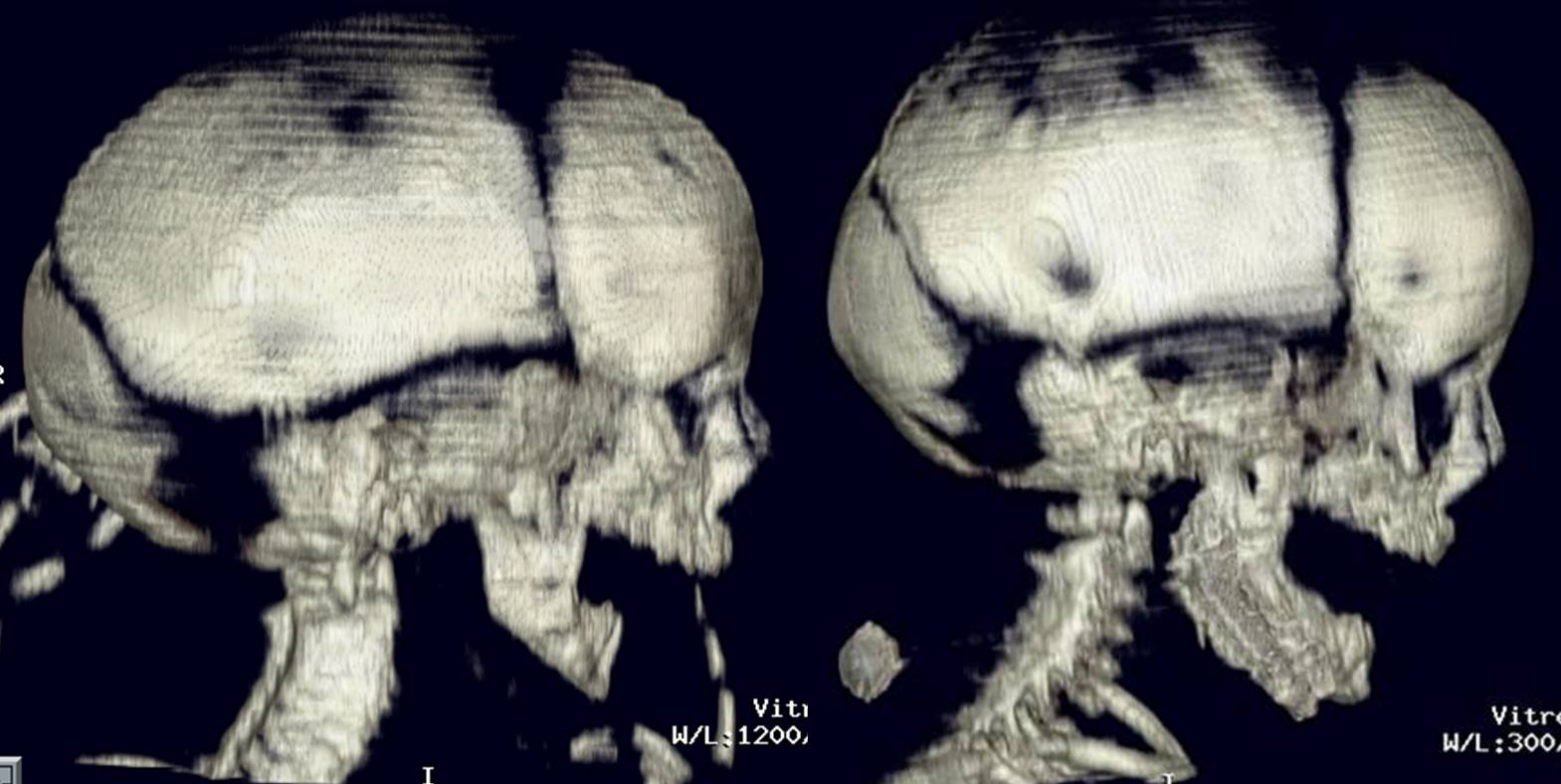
Three-dimensional CT scan taken previously and during the distraction period.
